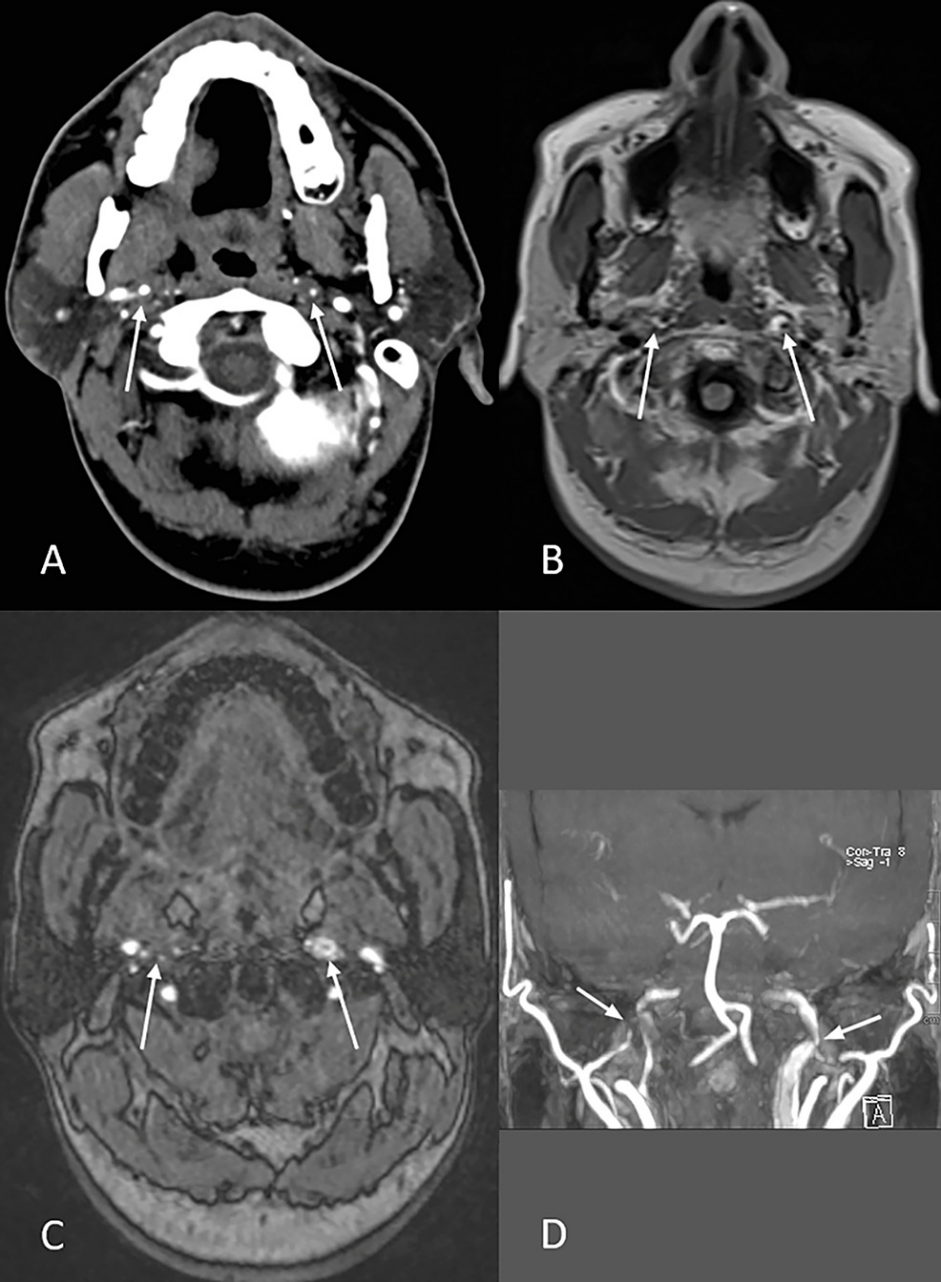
CT and MR angiograms showing Internal carotid artery dissection A: Axial CT image of the brain showing ill-defined circumferential hypodensity eccentrically surrounding bilateral cervical ICA; B: Axial T1W FLAIR image of the brain showing curvilinear hyperintensity at skull base around distal-most part of bilateral ICA just below the level of intrapetrous segments resulting in moderate to significant luminal stenosis of the lumen of bilateral ICA; C: Axial TOF image of the brain showing heterogeneously hypointense signal around bilateral ICA causing mild to moderate luminal stenosis; D: Coronal MR angiogram MIP image showing heterogeneously hyperintense signal around bilateral ICA. CT = Computed Tomography; MR = Magnetic Resonance; TOF = Time of Flight; ICA = Internal Carotid Artery; MIP = Maximum Intensity Projection; T1W FLAIR = T1-Weighted Fluid-Attenuated Inversion Recovery
